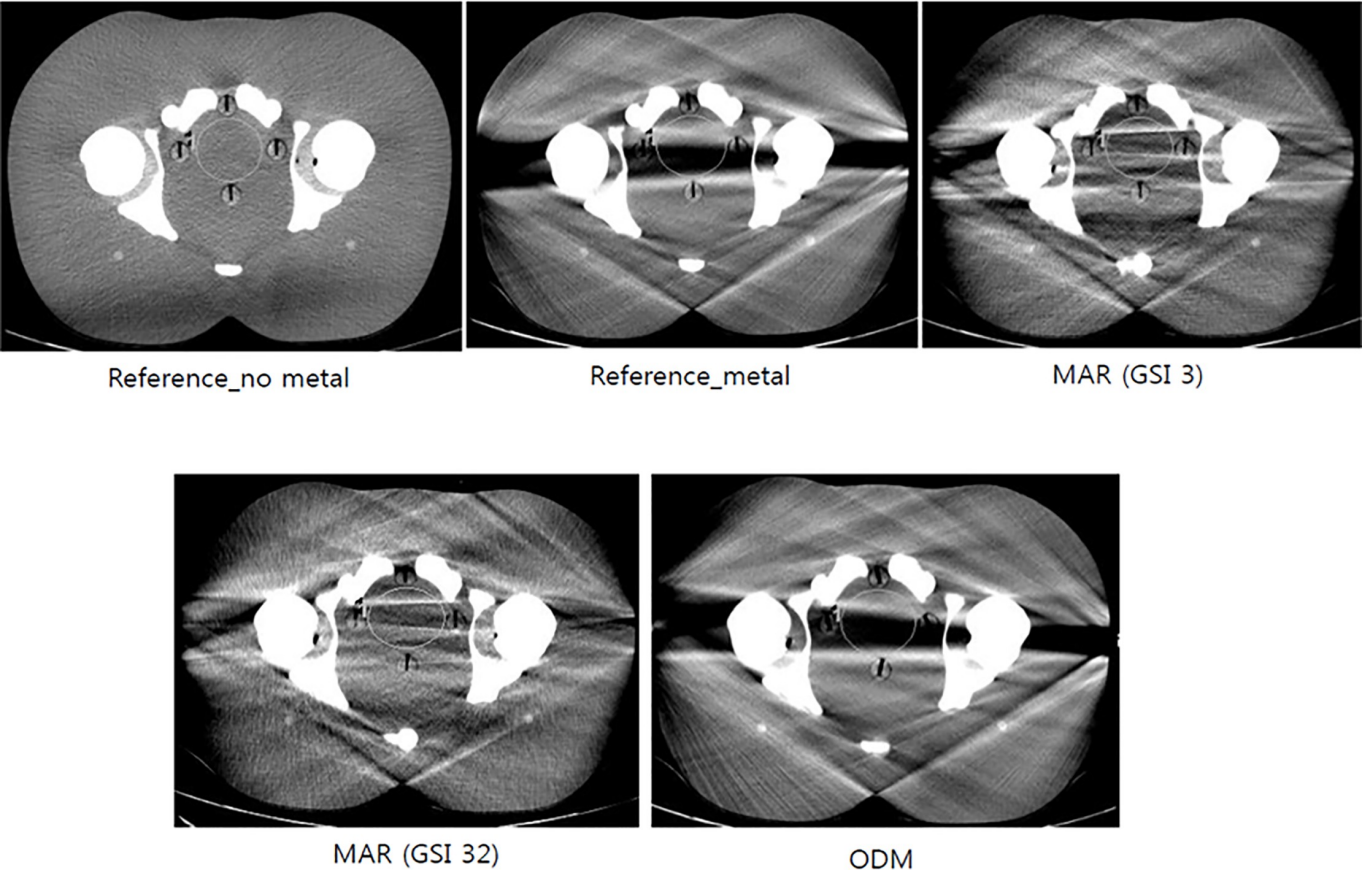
Region of Interest (ROI) for measuring the mean CT number and standard deviation (SD) of Hounsfield units (HU) in the pelvic cavity level.
